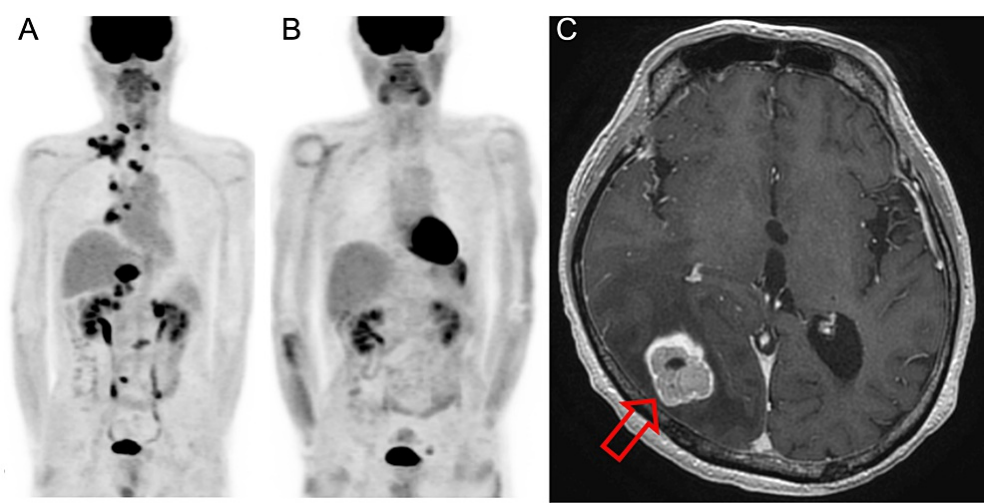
Case 1 (A) 18F-fluoro-D-deoxyglucose (FDG) positron emission tomography (PET)/CT before pembrolizumab treatment shows FDG uptake in the lower lobe of the right lung, multiple lymph nodes, multiple bone sites, and bilateral adrenal glands; (B) PET-CT after 48 cycles of pembrolizumab shows loss of uptake in all lesions; (C) Brain MRI after discontinuation of pembrolizumab shows a single brain metastasis in the right occipital lobe as indicated by the red arrow.
